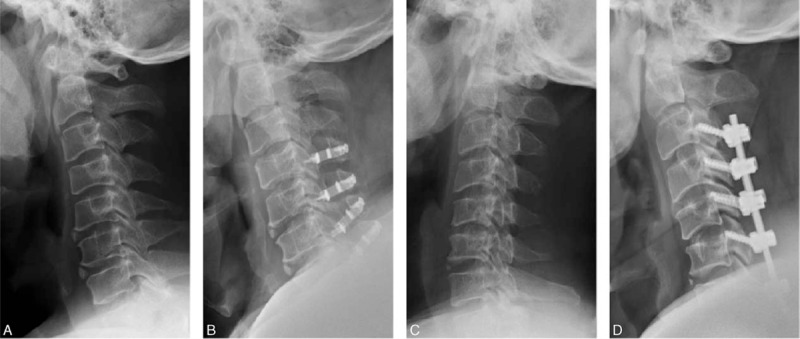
X-ray radiographs of the patients. (1A) Cervical laminoplasty preoperatively; (1B) cervical laminoplasty 1 year after operation. (2A) Cervical laminectomy and fusion preoperatively; (2B) cervical laminectomy and fusion 1 year after operation.
